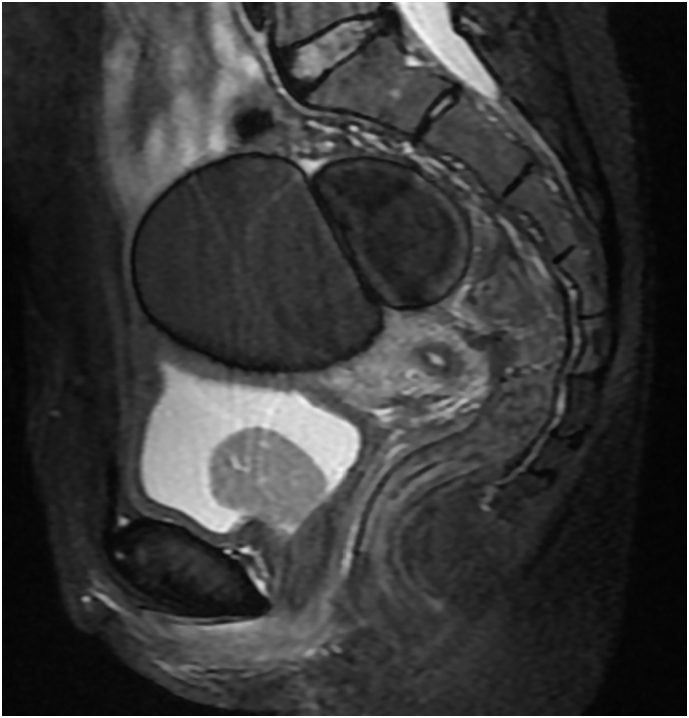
MRI showing the presence of an oval-shaped mass lesion arising from the base of the urinary bladder. The mass shows as low signal intensity on both T1 and T2 weighted images and enhances avidly following contrast medium administration.
